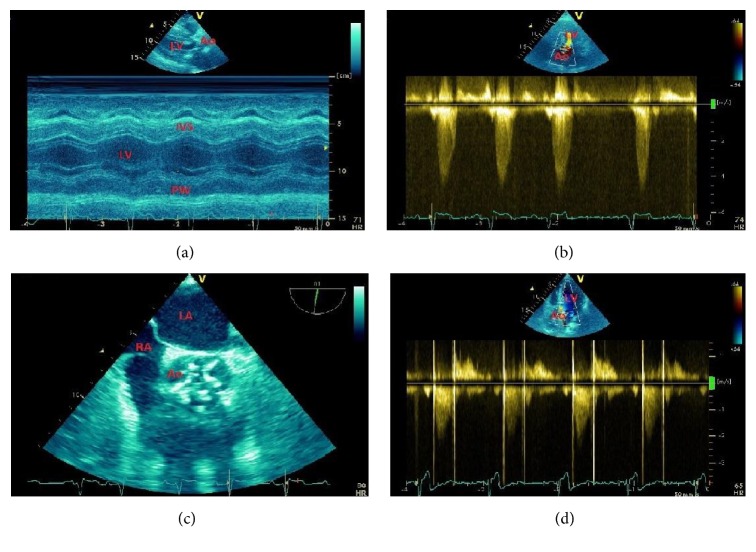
A 46-year-old male patient with valvar aortic stenosis (AS) and angina pectoris. Patient had aortic valve surgery. (a) Left ventricular hypertrophy from pressure afterload. (b) High transvalvular pressure gradient (81 mmHg peak, 44 mmHg mean) by color Doppler. (c) Reduced aortic valvar area (0.7 cm^2^) by TEE. (d) Aortic pressure gradient reduction (12 mmHg peak, 7 mmHg mean) after aortic valve surgery. Ao = aorta; IVS = interventricular septum; LA = left atrium; LV = left ventricle; PW = LV posterior wall; RA = right atrium.
